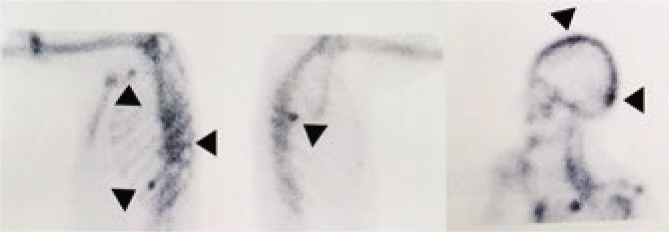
Bone scintigraphy depicting increased intake of Tc-99m in the skull, spine, anterior edge of the left 8th pleura, posterior edge of the right 5th pleura and the middle of the left clavicle (arrowheads), due to severe osteomalacia.
